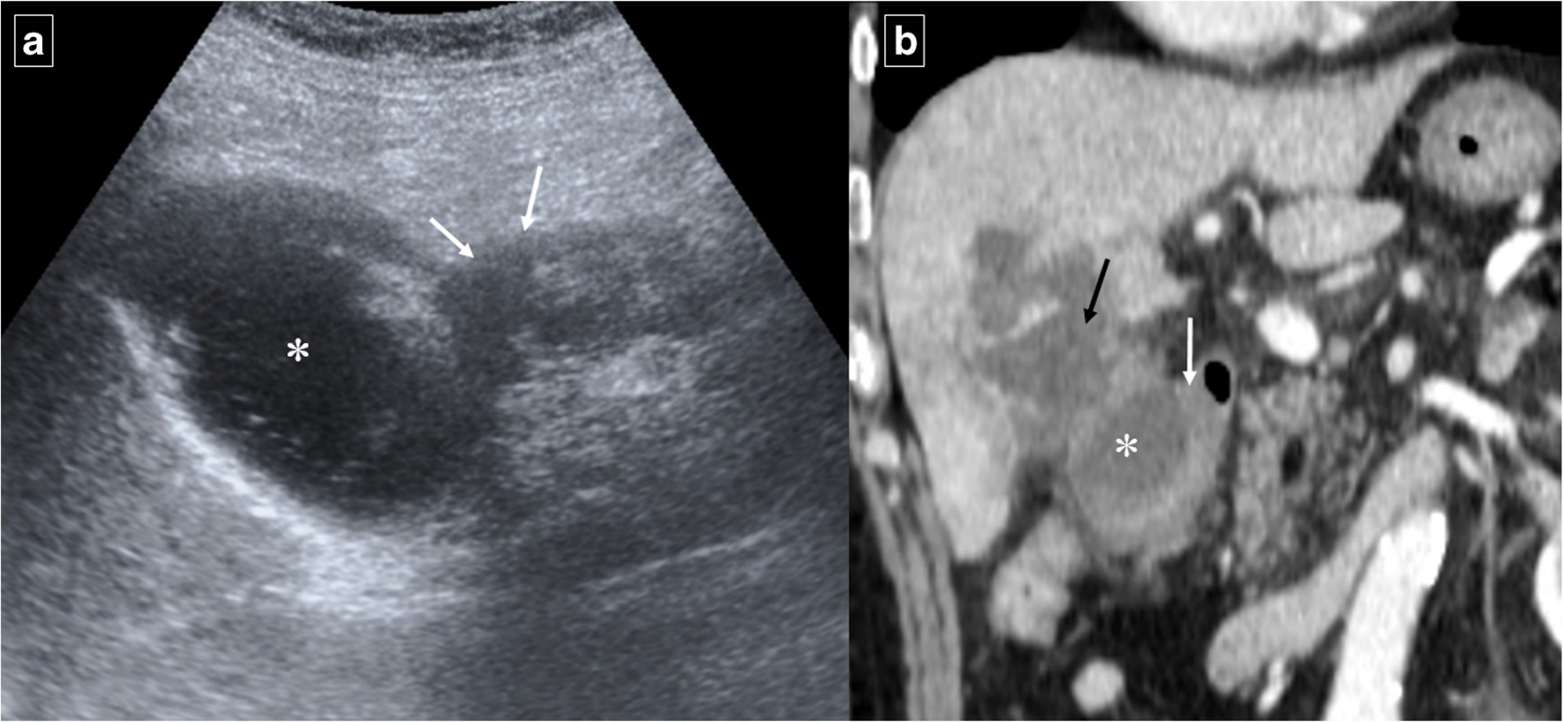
Acute cholecystitis in a 54-year-old man. a Ultrasonography identified an enlargement of the lateral wall of the duodenum (white arrows) that was accompanied by a periduodenal fluid collection (*). b Reformatted coronal contrast-enhanced CT image shows a distended gallbladder with inaccurate margins (black arrow) and confirms the presence of a periduodenal fluid collection (*). Also note the secondary duodenal wall involvement (white arrow)
